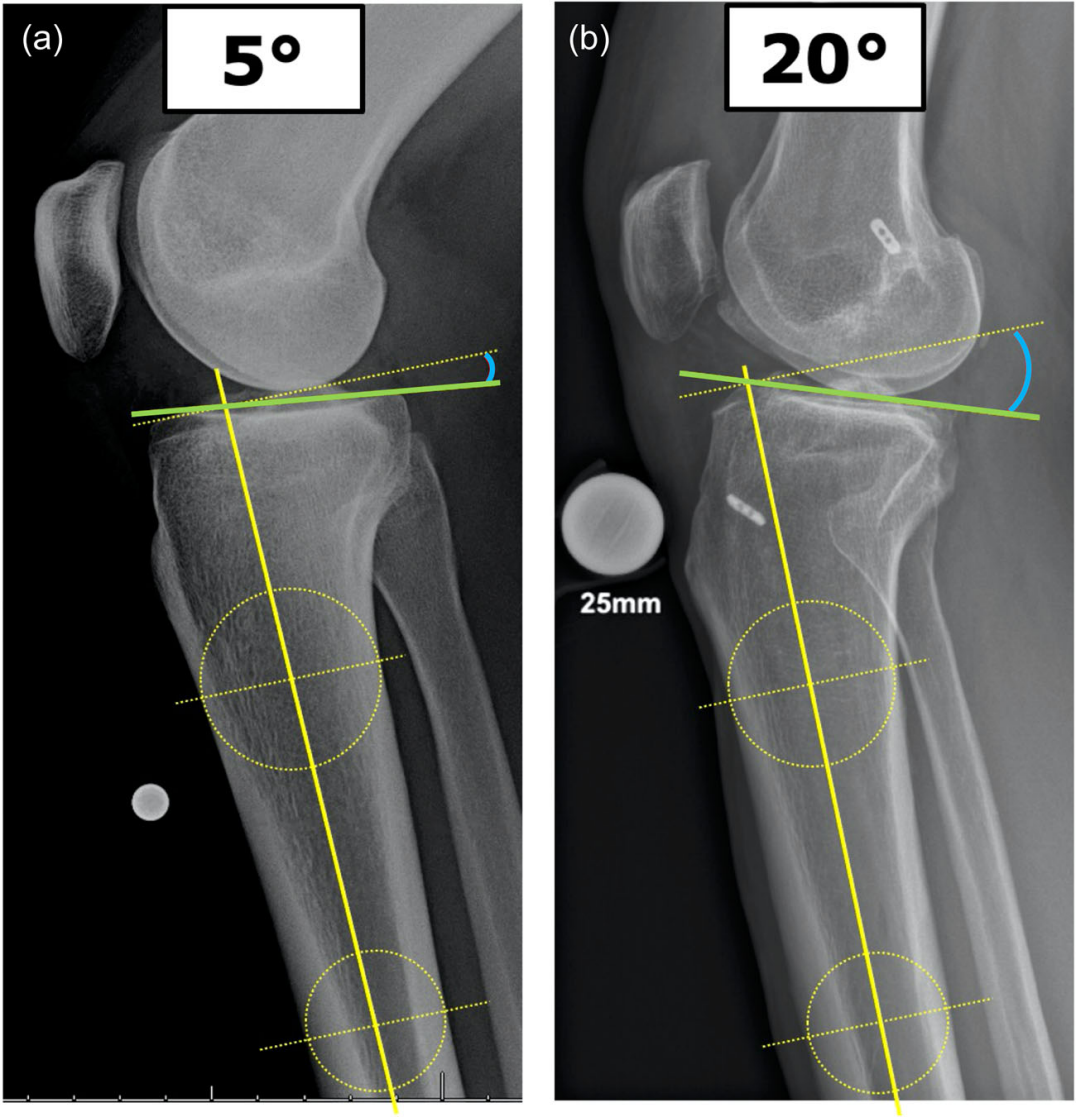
The posterior tibial slope (PTS) can be determined based on lateral knee radiographs. Two concentric circles (yellow dotted circles) are superimposed on the tibia approximately 5 and 10 cm distal to the tibiofemoral joint line. A vertical line transecting the centres of the two circles is drawn to determine the anatomic axis of the tibia (vertical yellow line). The angle (blue line) between a line drawn perpendicular to the anatomic tibial axis (yellow dotted line) and another drawn tangential to the tibial plateau (green line) corresponds to the magnitude of the PTS. (a) A PTS of 5° is considered normal and does not increase the risk of primary ACL injury or ACL revision. (b) A PTS of 20° may lead to unfavourable knee kinematics and greater anteroposterior tibial translation, which leads to an increased risk of both primary ACL injury and ACL revision. ACL, anterior cruciate ligament.
